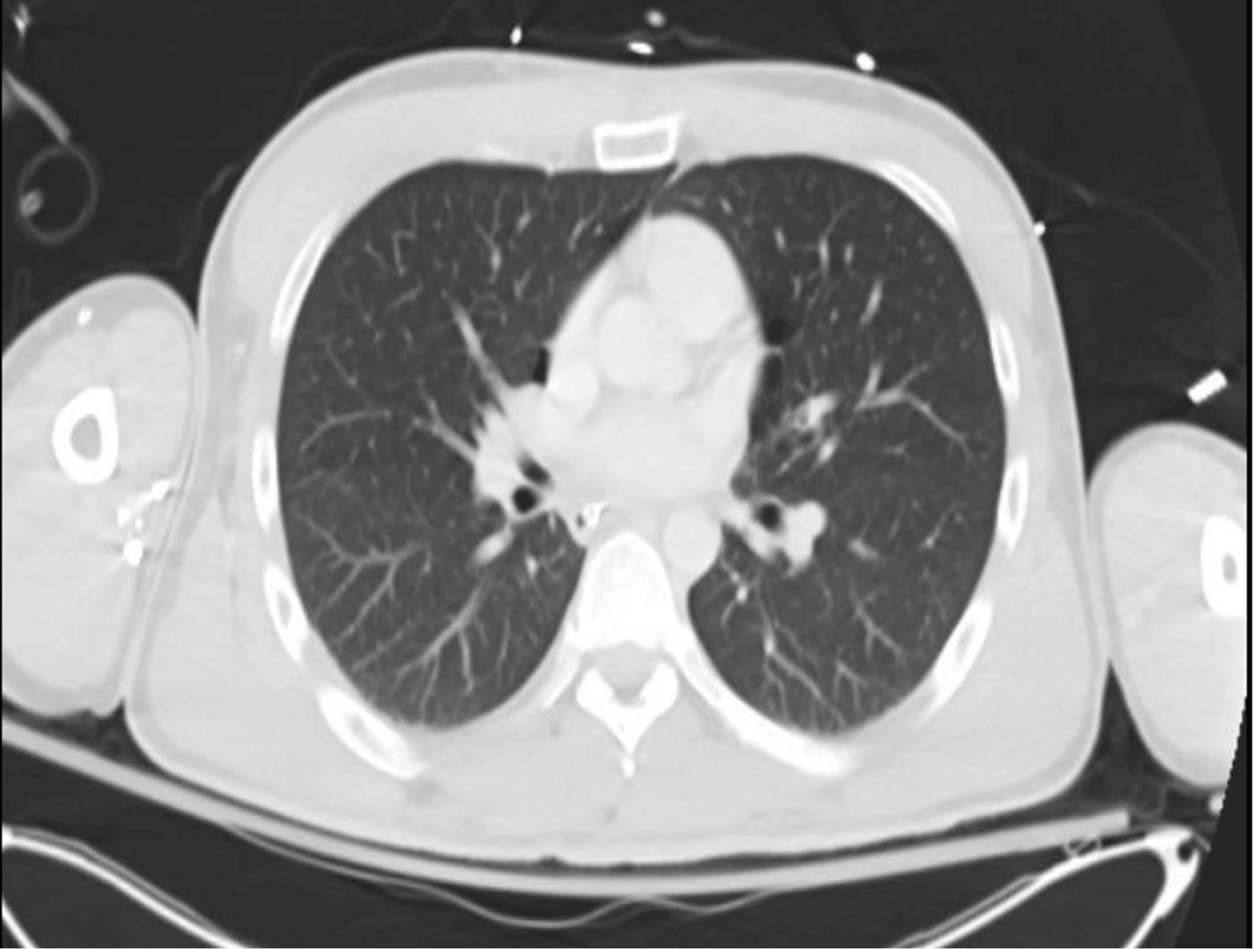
Non-contrast computed tomography of the chest demonstrating no acute abnormalities.
